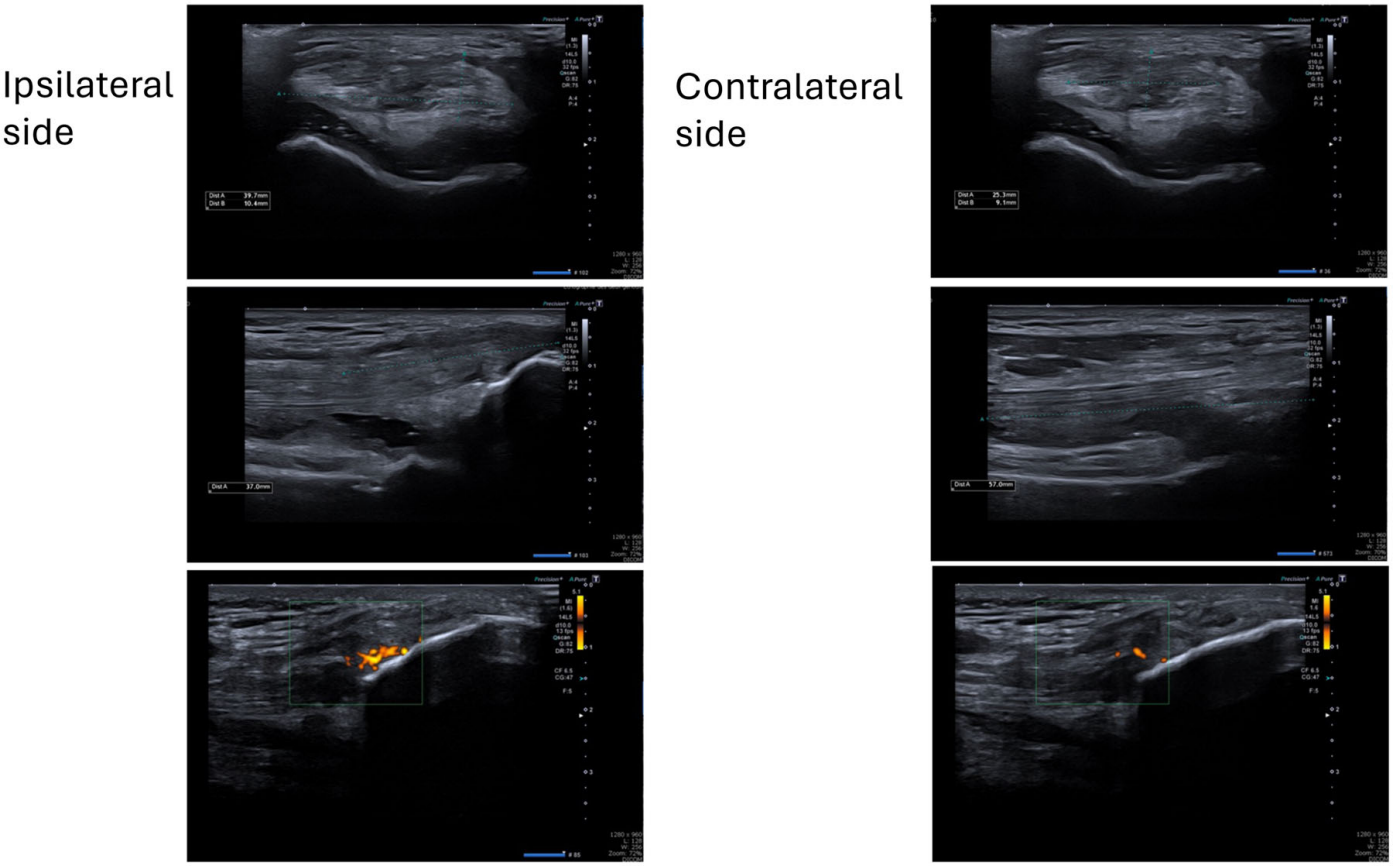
Ultrasound evaluation of the ipsi‐ and contra‐lateral knee.
